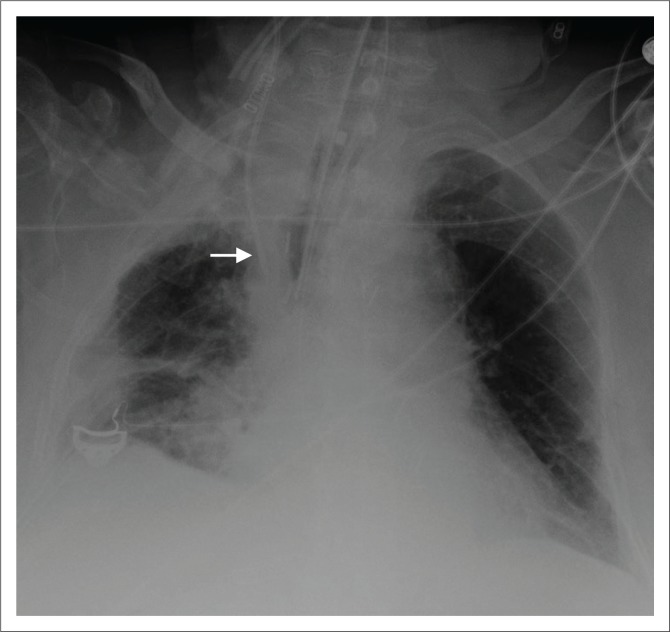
Magnified chest radiograph showing a widened superior mediastinum secondary to central venous catheter induced haematoma.
